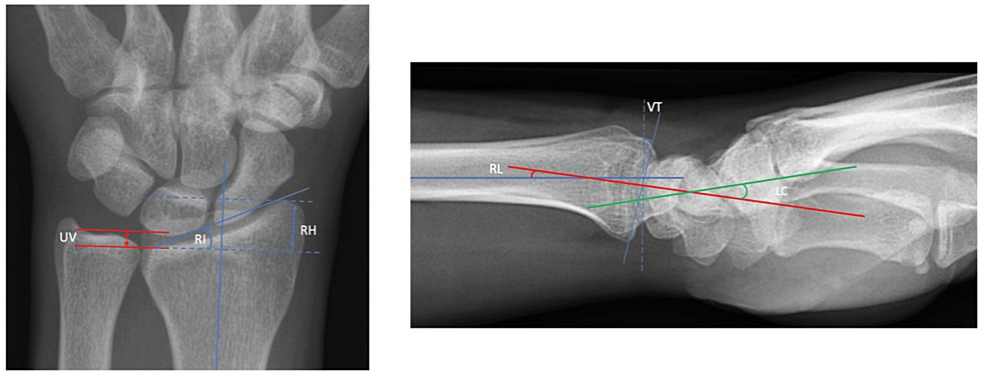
Established radiographic parameters Left: Posteroanterior radiograph ulnar variance (UV) = -2mm to +2mm; radial inclination (RI) = 22 degrees; radial height (RH) = 12mm; right: Volar tilt (VT) = 11 degrees; radiolunate angle (RL) = 10 +/- 6 degrees; lunocapitate angle (LC) = 0 +/- 12 degrees
